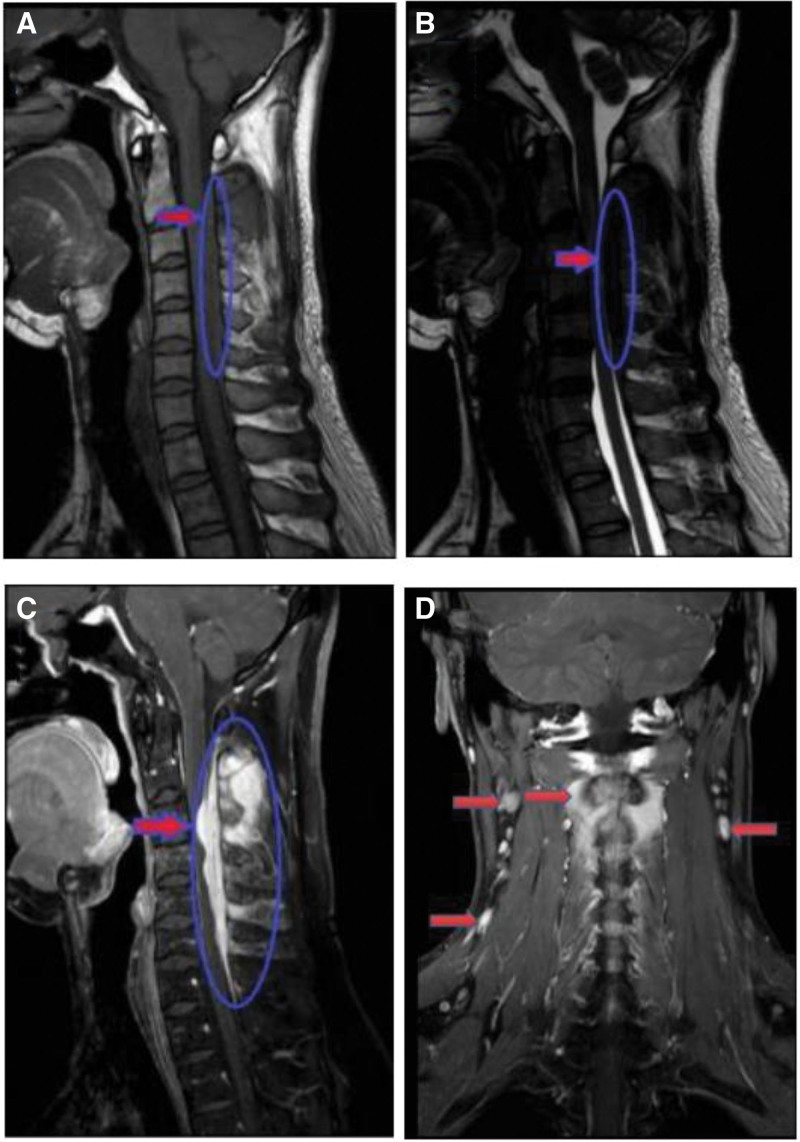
Contrast-enhanced magnetic resonance images of the spine. The lesion is hypointense on T1-weighted (A) and T2-weighted images (B). After intravenous administration of gadolinium, the tumor showed obvious peripheral enhancement and a tendency to compress the spinal cord anteriorly (C). Soft-issue-occupying lesions in the posterior neck with little cancellous bone destruction can be seen at the C2 and C3 levels. The lymph nodes in the neck are enlarged on both sides, and some lymph nodes are slightly enlarged (D).
